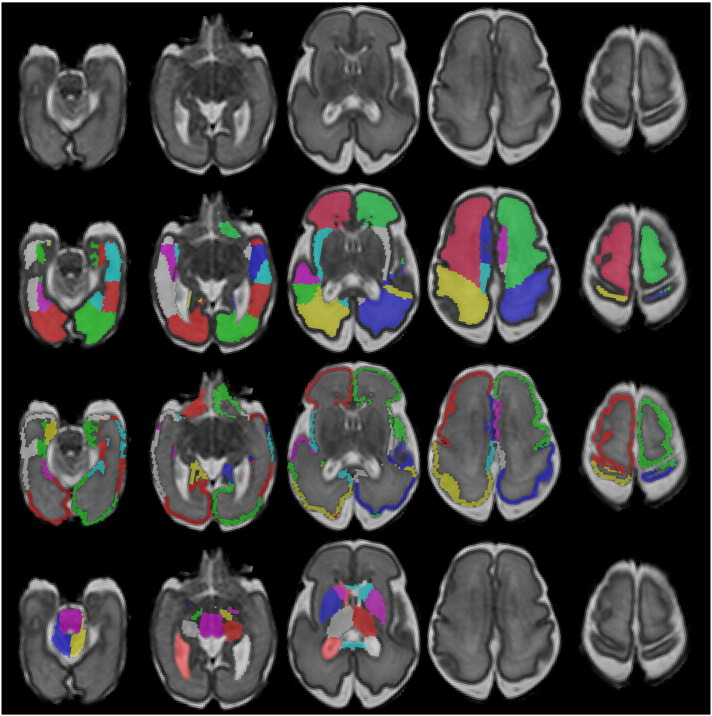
Example segmentation of a neonatal MRI acquired at 28 weeks PMA at scan with the 82 labels overlaid (second row: WM labels, third row: CGM labels, fourth row: subcortical GM labels and ventricles).
